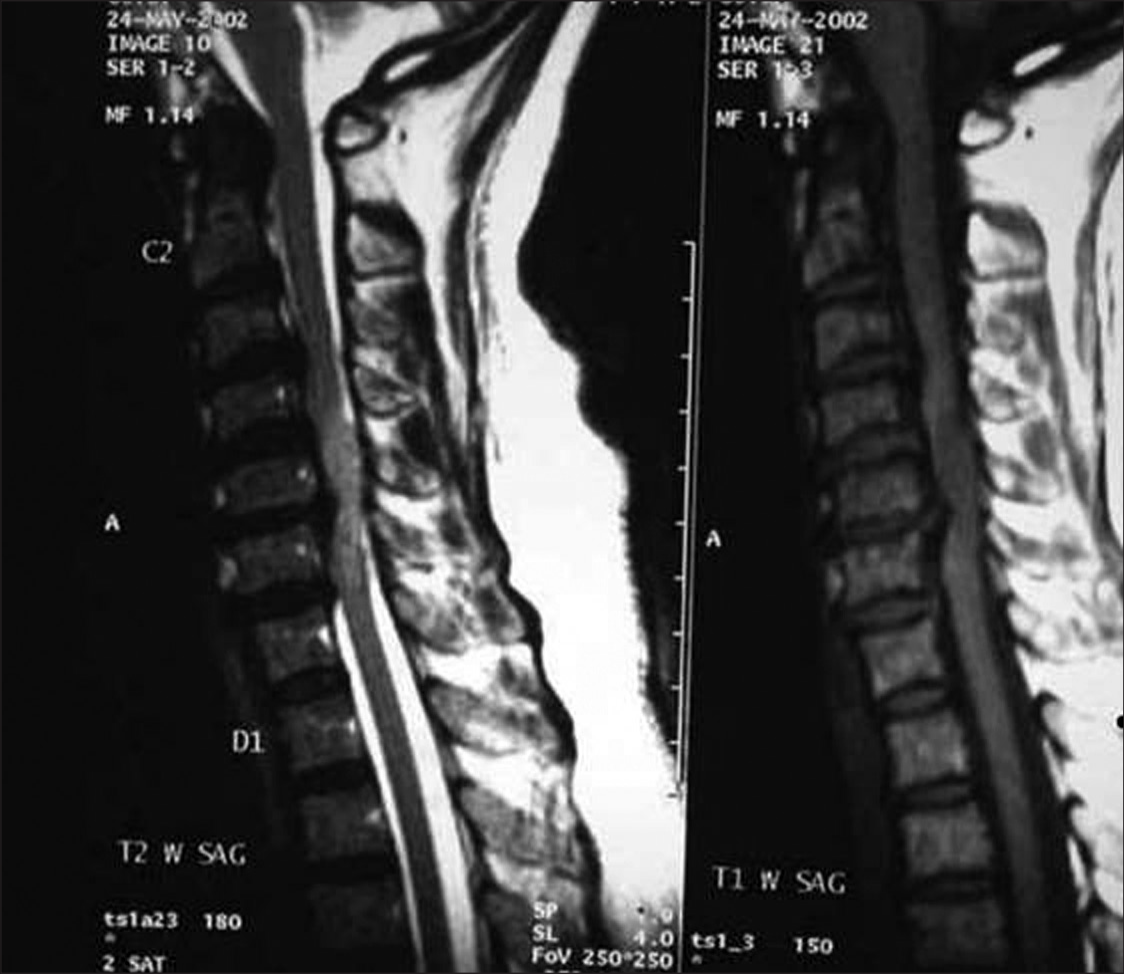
Preoperative sagittal TI T2 MRI images show disc herniation and the tumor
